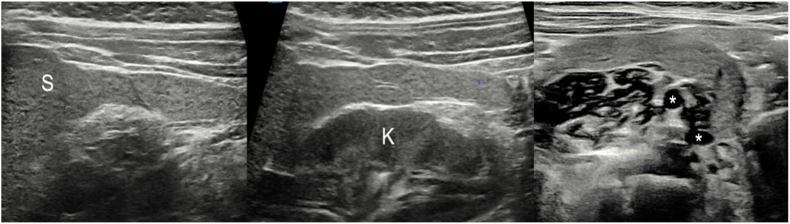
Sequential longitudinal ultrasound images of continuous splenogonadal fusion. Note the tail-like structure with splenic echotexture originating from a normally located spleen (S), traversing the left paracolic gutter laterally to the left kidney (K), and finally embracing the left ovary, which contains small follicles or cysts (asterisks).
